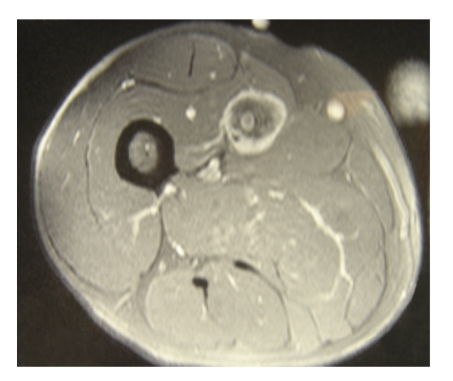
MRI picture of Leiomyosarcoma arising from the femoral vein.
